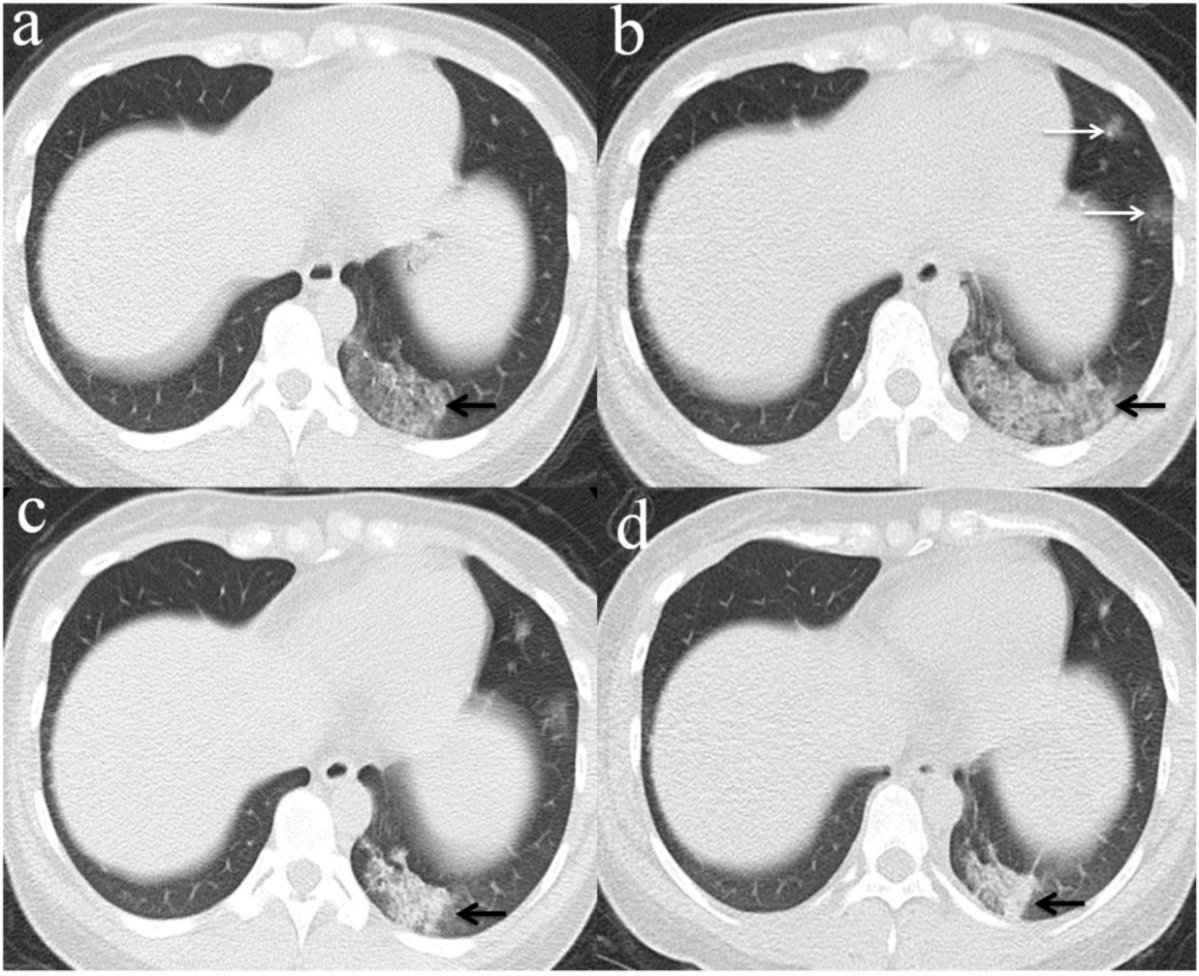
Evolution of chest CT imaging findings in a 30-year-old female patient presenting with fever (range:38.1-39℃), cough and expectoration for four days. (a)6 days after initial symptoms, subpleural GGO with partial consolidation was presented in the left lower lobe(black arrow).While, the patient's initial RT-PCR was negative; (b) day 11, there was an enlarged region of GGO and consolidation(black arrow),and was demonstrated new GGO appeared in other lesions of left lobe(white arrows).One day later, swab test turned positive for SARS-CoV-2; (c) day 16, the overall range of lesions was smaller than that of day 11, but the subpleural consolidation were more frequent(black arrow);(d) day 22, continued resolution with consolidation(black arrow) and other lesions of left lobe demonstrated remission.
